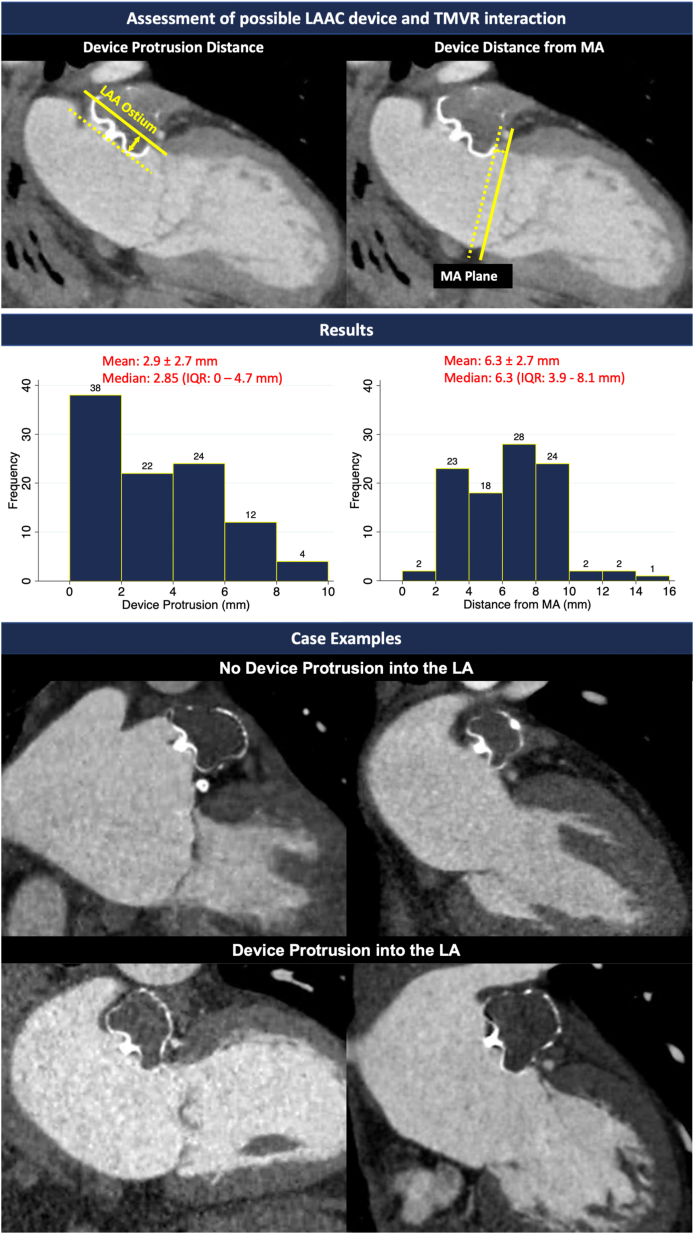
Computed Tomography Assessment of WATCHMAN FLX Device Protrusion From Left Atrial Appendage With Case Examples LA = left atrium; LAA = left atrial appendage; LAAC = left atrial appendage closure; MA = mitral annulus; TMVR = transcatheter mitral valve replacement.
